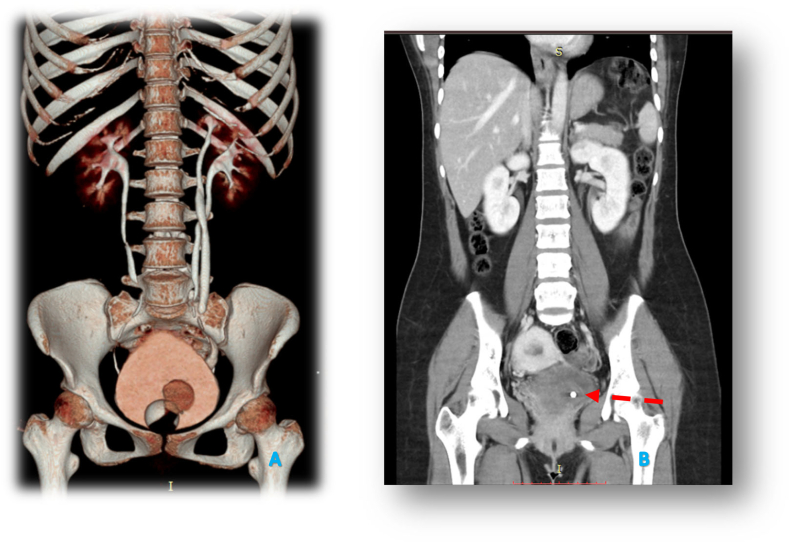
A and B. CT IVU in patients showing the imaging of left intravesical ureterocele left the complete double system and radiopaque shadow on the left UVJ with the impression of stone (shown by red arrows). (For interpretation of the references to colour in this figure legend, the reader is referred to the web version of this article.)
